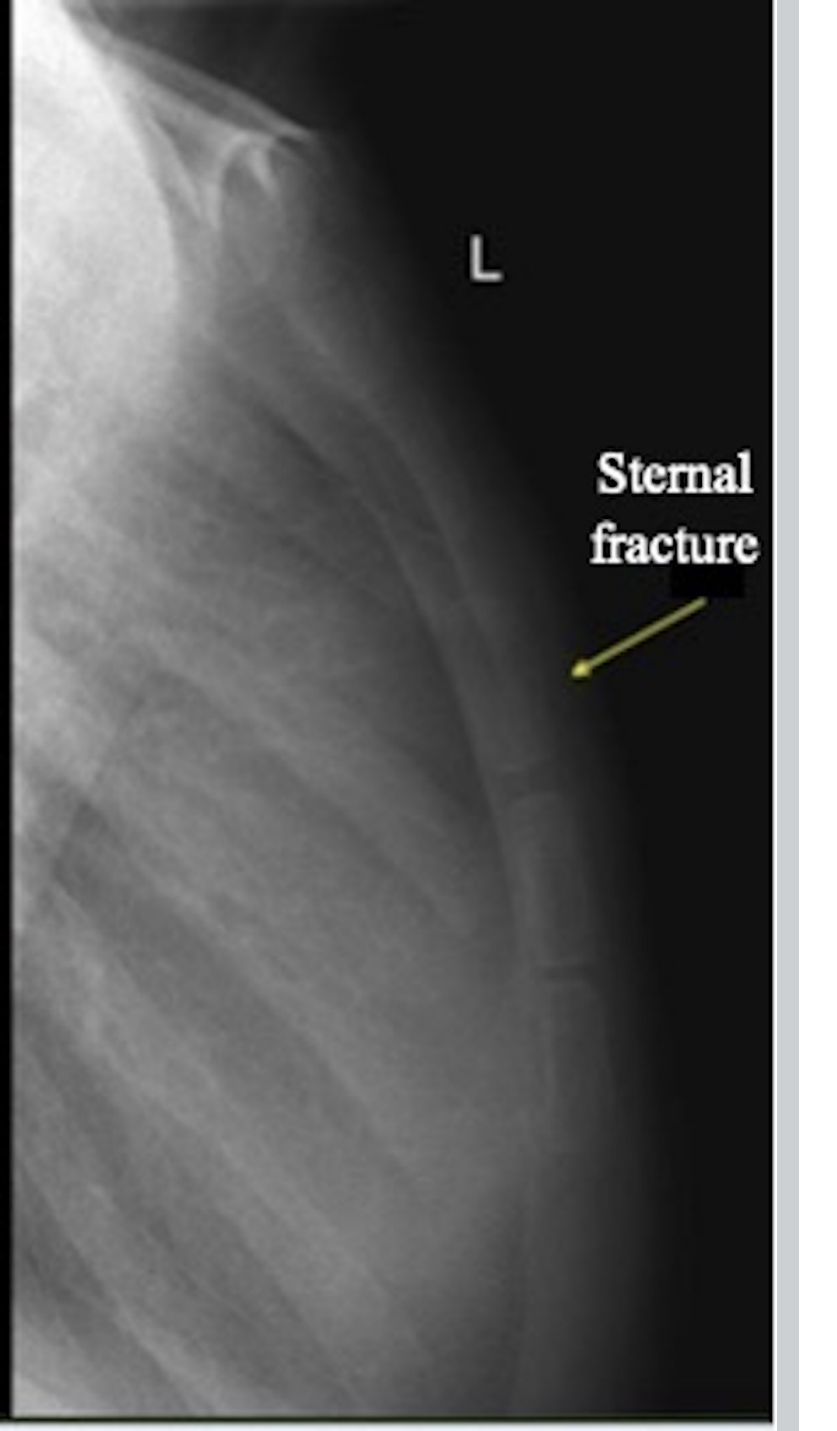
Plain radiograph of the sternum. A discontinuation of the radiopaque structure was detected on the sternal body, suggesting a sternal fracture (arrow).
